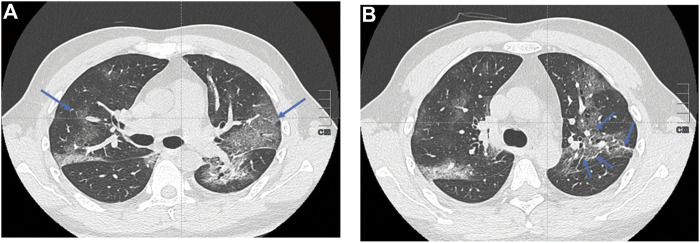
Chest computed tomography images of patients with COVID-19 pneumonia (A) shows Ground-glass opacities (blue arrows) (B) shows confluent crazy-paving pattern and consolidation opacities: secondary appearance of intralobular reticulations (blue arrows).
